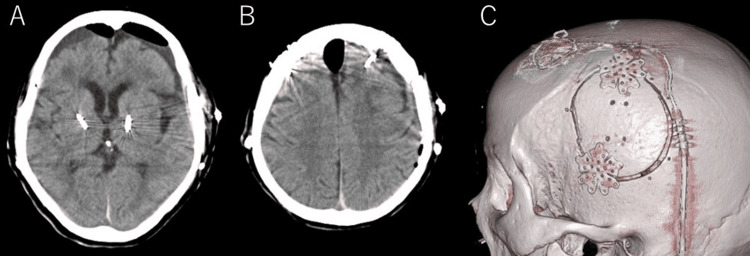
Postoperative head computed tomography (CT) and three-dimensional CT (3DCT) (A, B) Postoperative head CT showing sufficient hematoma removal and improved midline shift after the procedure. (C) 3DCT showing the cranial bone after the procedure. A linear skin incision was made parallel to the deep brain stimulation lead, and a small craniotomy was performed.
